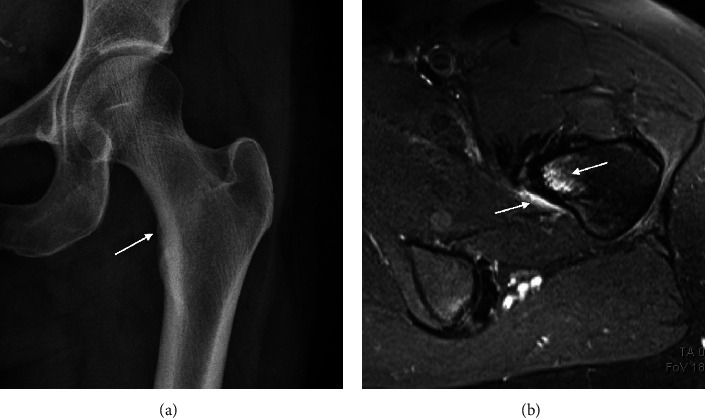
(a) X-ray of a 37-year-old female showing cortical thickening (arrow) along the medial femoral neck inferiorly in keeping with a stress reaction. (b) Axial T2 FS MRI showing marrow edema and periosteal edema, confirming stress reaction.
